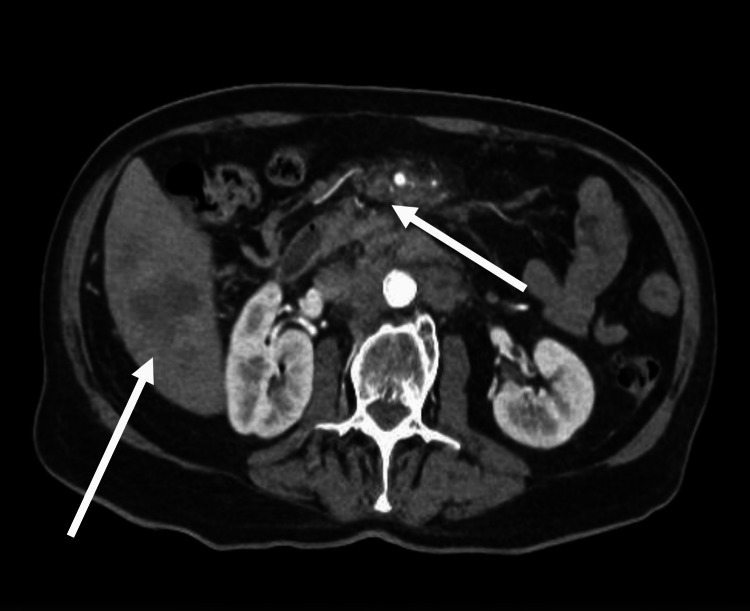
Computed Tomography of the abdomen and pelvis showing primary pancreatic malignancy with liver metastasis. Axial contrast-enhanced CT image showing a pancreatic mass with associated hepatic metastases. (Image obtained from the hospital PACS system with patient consent.) Arrows indicate liver metastases and pancreatic malignancy.
